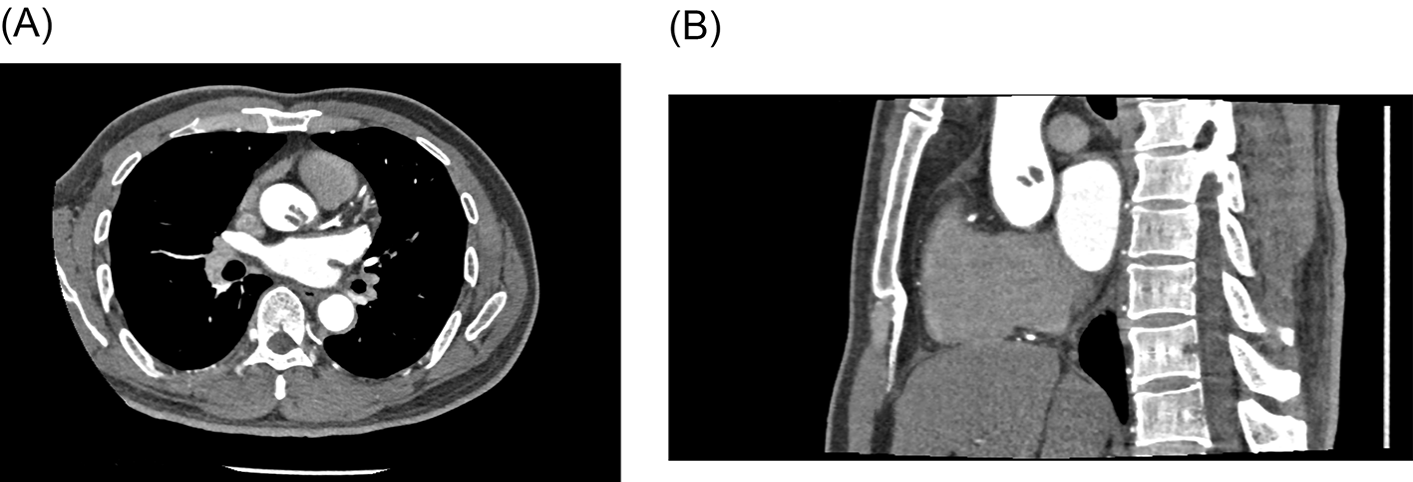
Coronary CTA images in (A) axial and (B) sagittal planes showing a lobulated filling defect suggestive of a thrombus, located in the proximal portion of ascending aorta. The thrombus occurs just above the left coronary ostium, closed to the sinotubular junction and the sinus of Valsalva. No abnormality was found in the coronary artery
